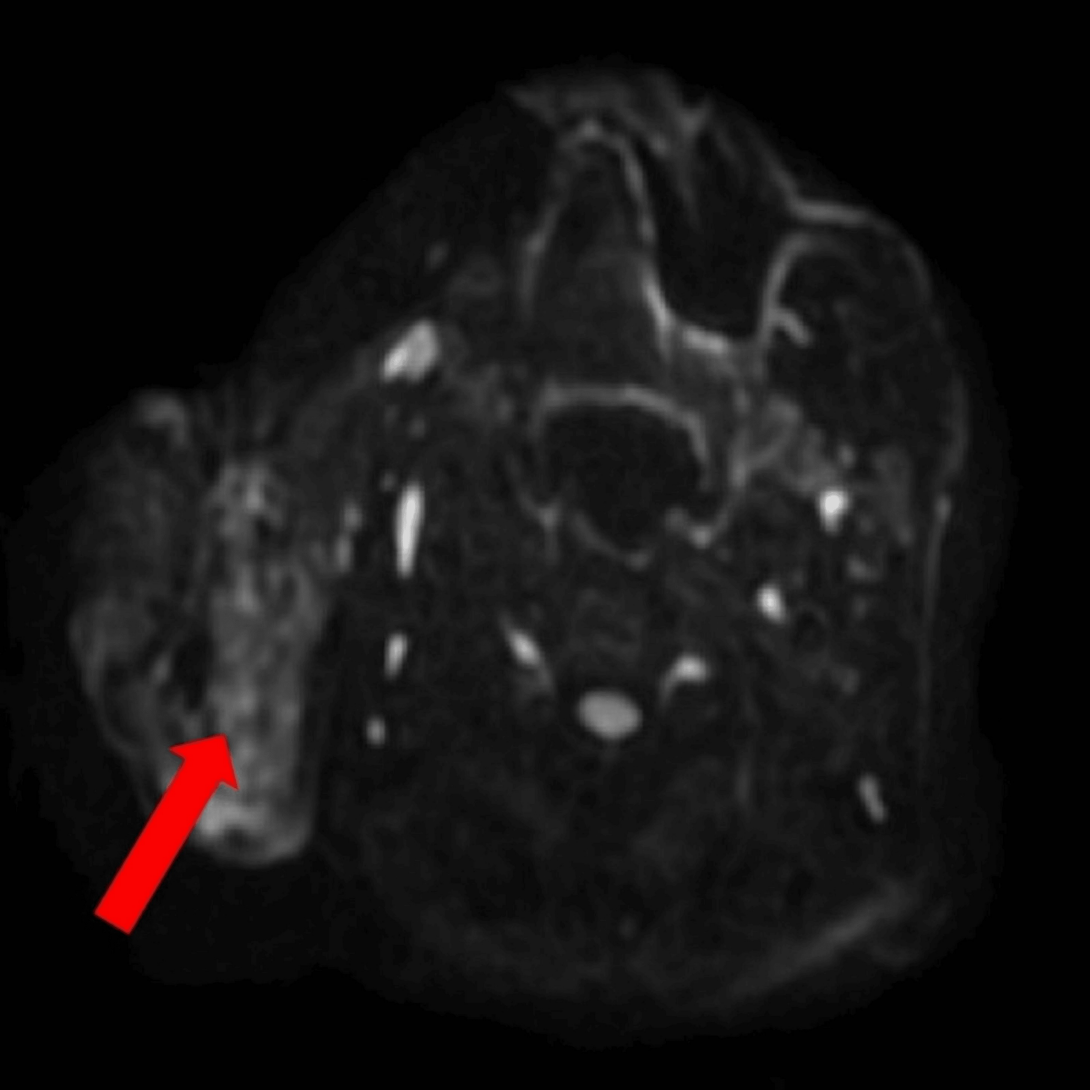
DWI Axial DWI demonstrating a hyperintense lesion in the right parotid gland (red arrow), consistent with an inflammatory process. No cystic, necrotic, or diffusion-restrictive features are noted. DWI: diffusion-weighted imaging
